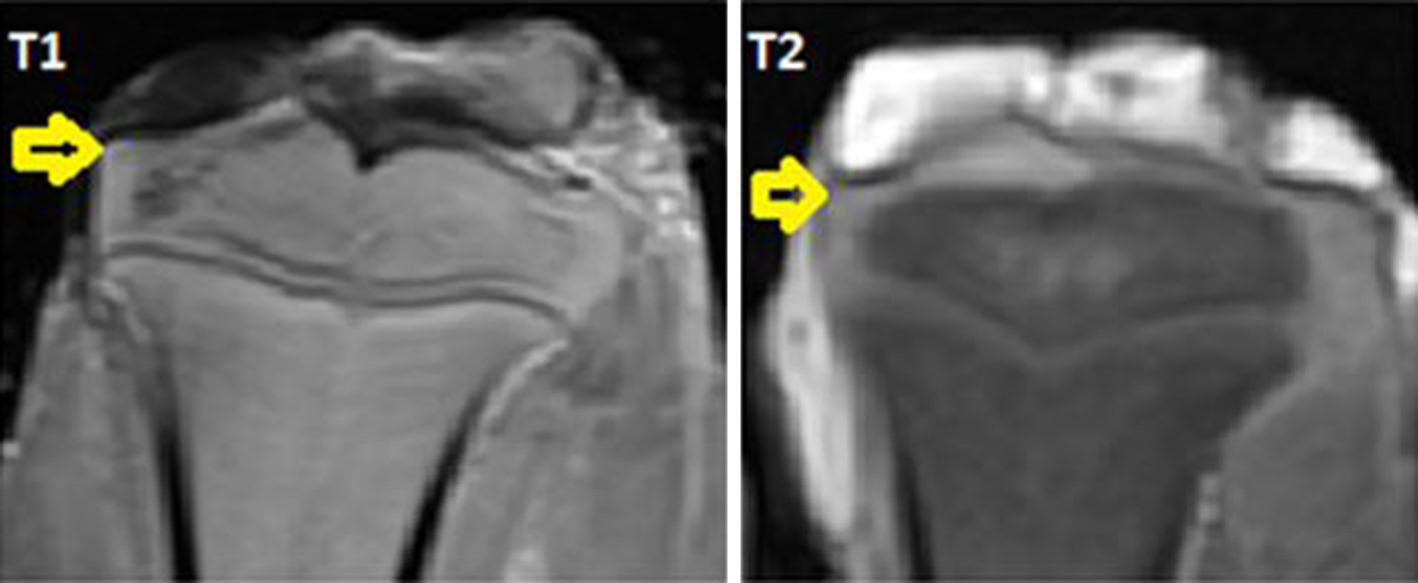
Positive controls with ablated joint cartilage. On magnetic resonance imaging (MRI), hyper-intense changes can be observed in the intentionally ablated joint cartilage (*arrows*)
